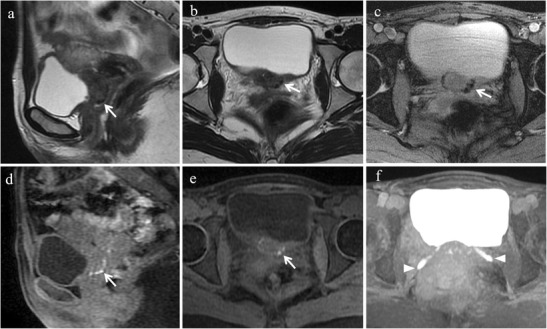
Bladder endometriosis with intrinsic involvement in a 35-year-old woman with dysuria, hematuria and urinary incontinence during menses. (a) Sagittal and (b) oblique axial T2-weighted images show a mural mass with low signal intensity infiltrating the posterior bladder wall (white arrows). On (c) axial T2* image punctate signal voids due to hemosiderin deposition can be seen along the borders of the lesion (white arrow). On (d) sagittal and (e) oblique axial fat-suppressed T1-weighted images the implant displays intermediate signal intensity with spots of high signal intensity, representing hemorrhagic content (white arrows). (f) Oblique axial MIP image (acquired about 10 min after contrast administration) demonstrates the relationship between the lesion and the ureters (white arrowheads)
